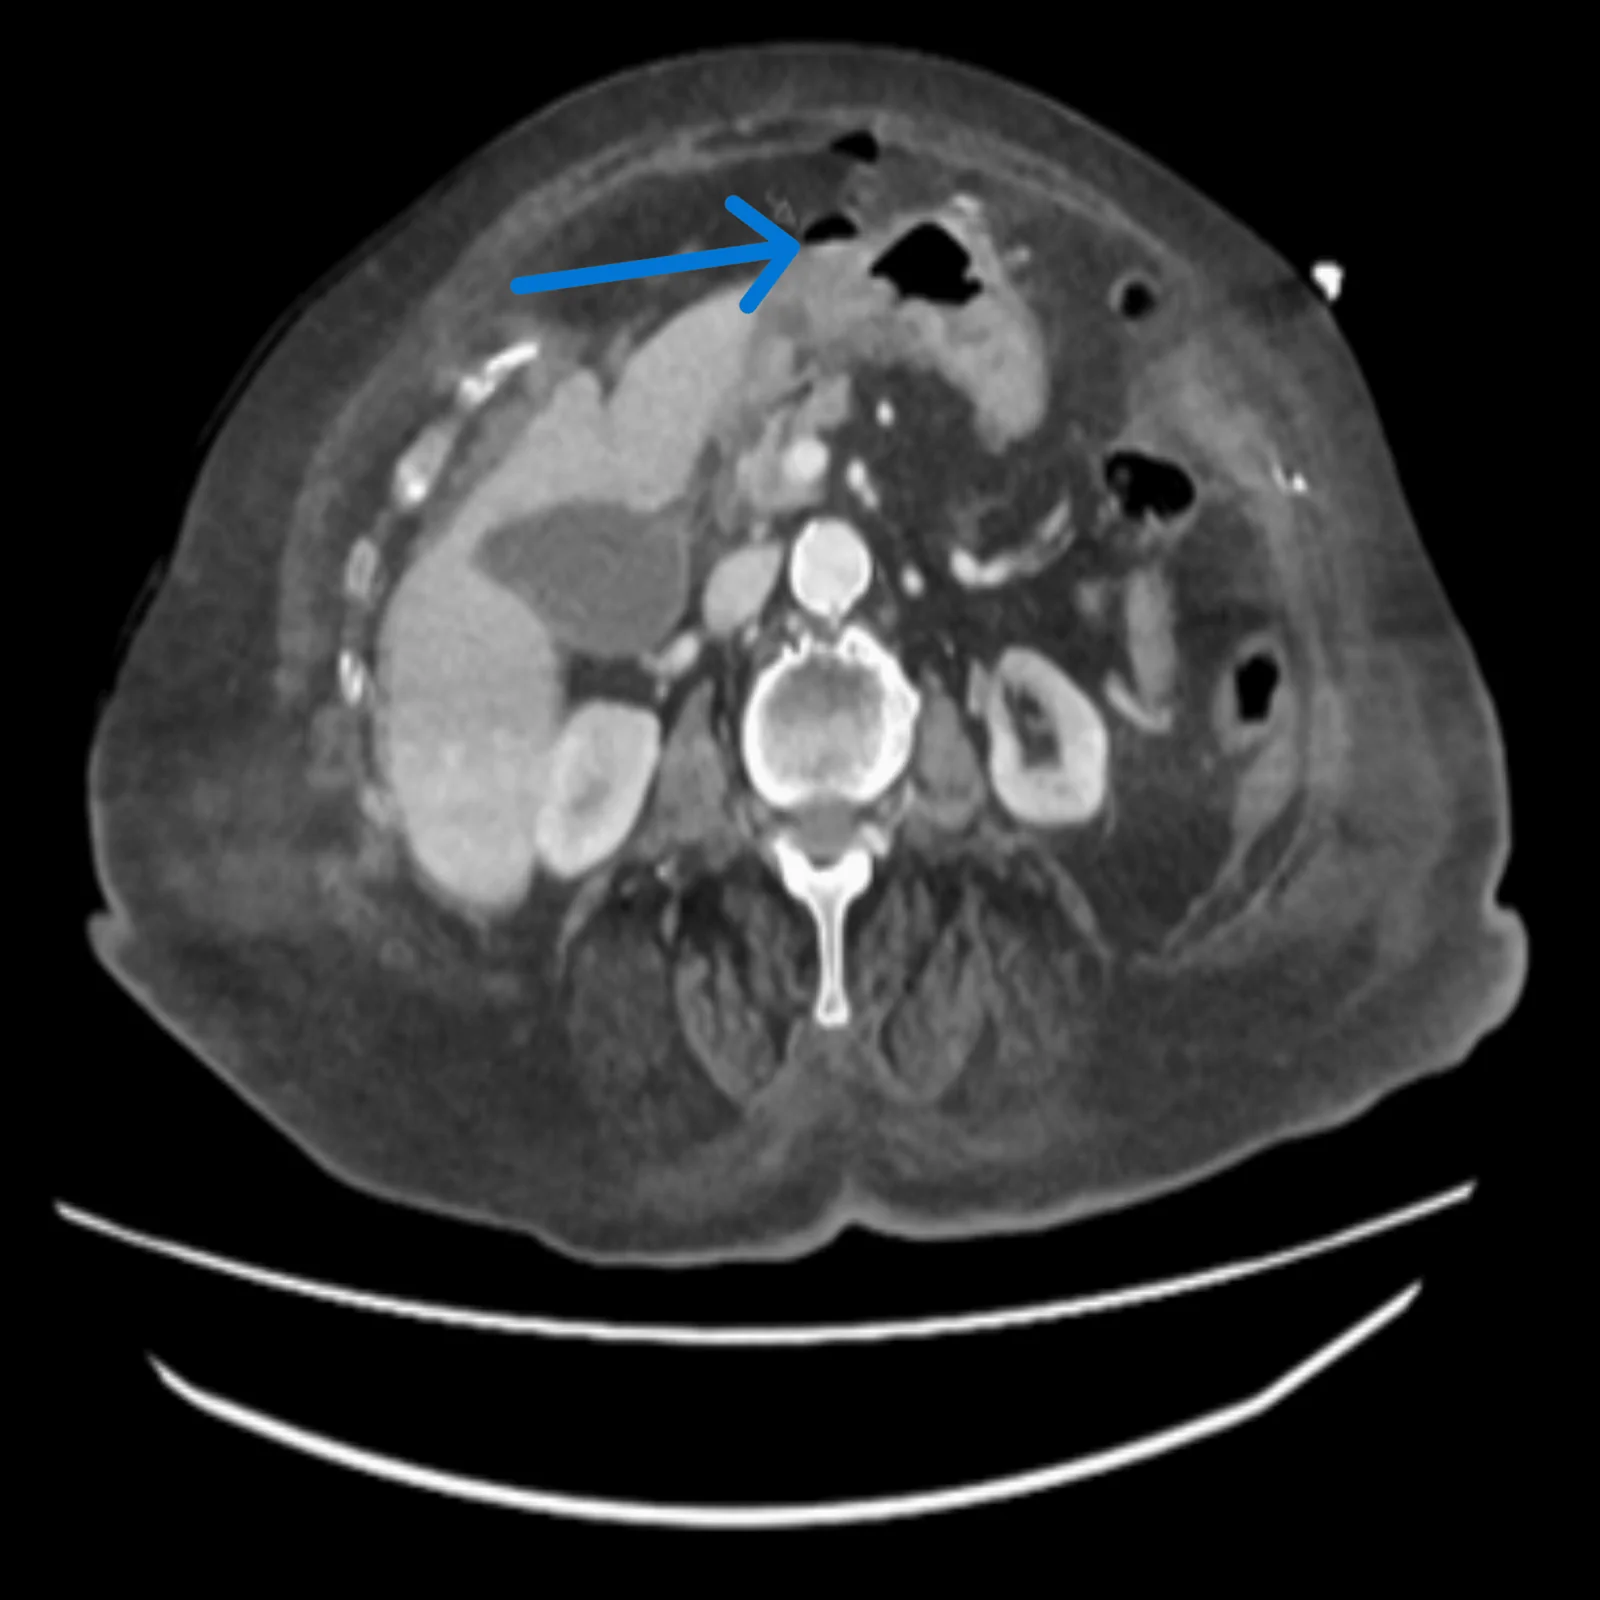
Computed tomography of the abdomen (non-contrast) The arrow indicates extraluminal gas adjacent to the greater curvature of the stomach suggesting possible pneumoperitoneum.
